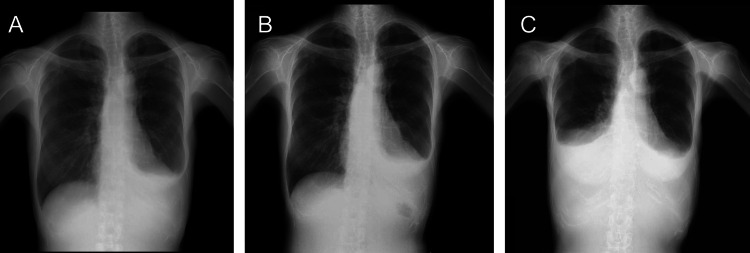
Chest radiography images Chest radiography images taken (A) 25 years, (B) 27 years, and (C) 33 years after thoracic radiation therapy for Hodgkin lymphoma.
